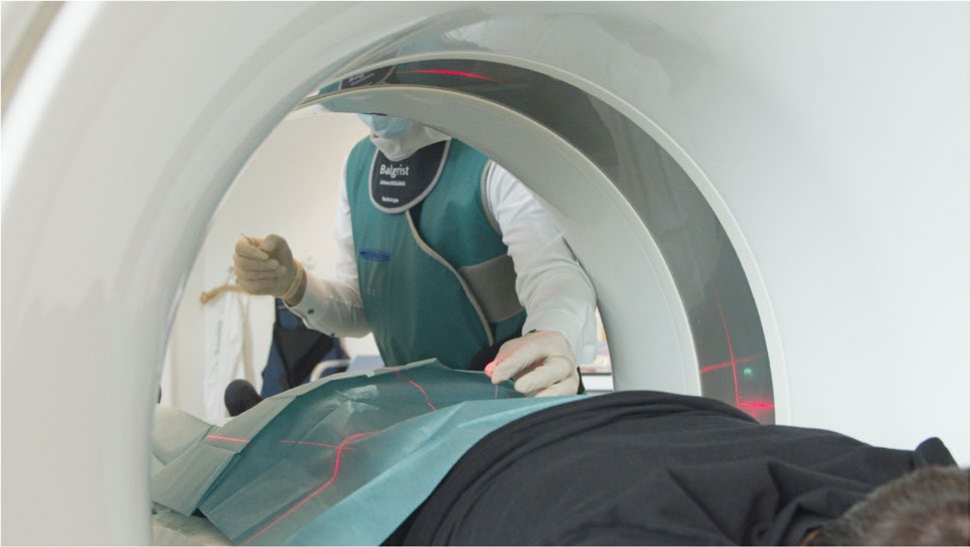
Radiologist performing a CT-guided corticosteroid injection in the lumbar spine. Patient placed in prone position with sterile sheet placed on the back. Laser navigation is used for precise needle placement
